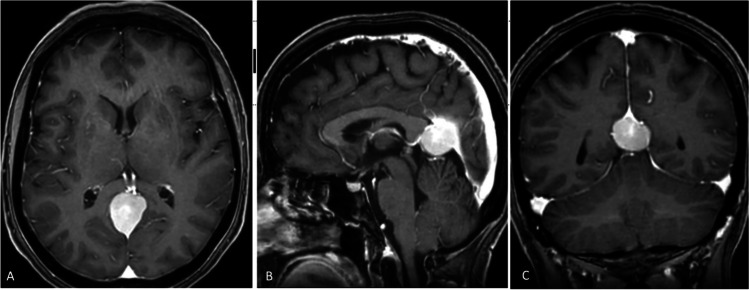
A 57-year-old female without any past medical history presented to our institution with headache and neck pain for the last 6 months. She did not have any other neurological signs or symptoms. A full neurological examination was within normal limits. Cranio-cervical MRI was performed, which revealed a 30 × 25 × 20 mm extra-axial tumor compatible with meningioma. A axial, B sagittal, and C coronal T1 contrast enhanced MRI. The tumor was located at the anterior part of falcotentorial junction projecting into the pineal region and found displacing the deep venous system upwards. Notably, the inferior sagittal sinus, the vein of Galen and the straight sinus were patent. At surgery we confirmed the fact that the primary origin of the tumor was from the tentorium extending to the falcotentorial junction, posterior part of inferior sagittal sinus and anterior part of the straight sinus (type T3 Yasargil’s classification) [8]
